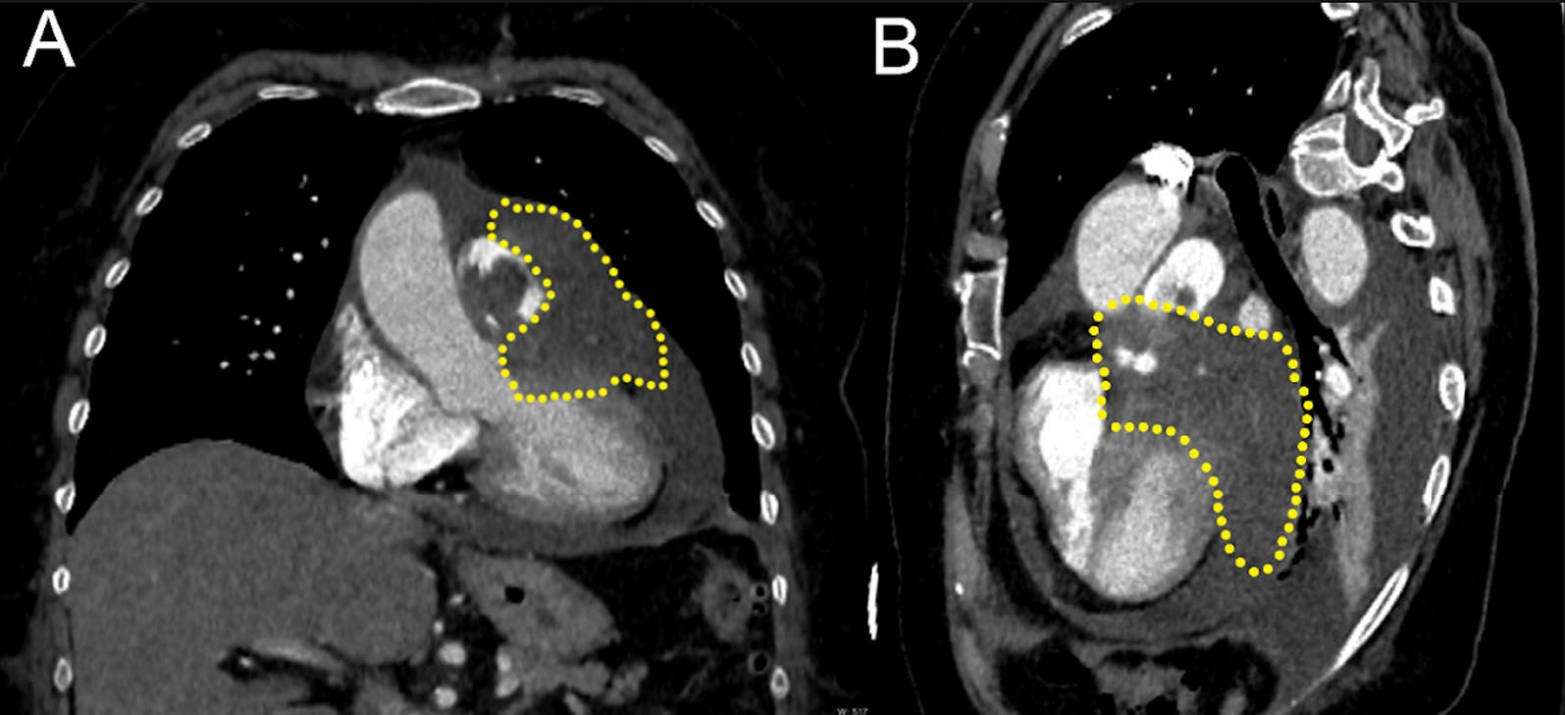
Chest tomography. A, B A Coronal and oblique views on a CT, confirming the location of the cardiac tumor
